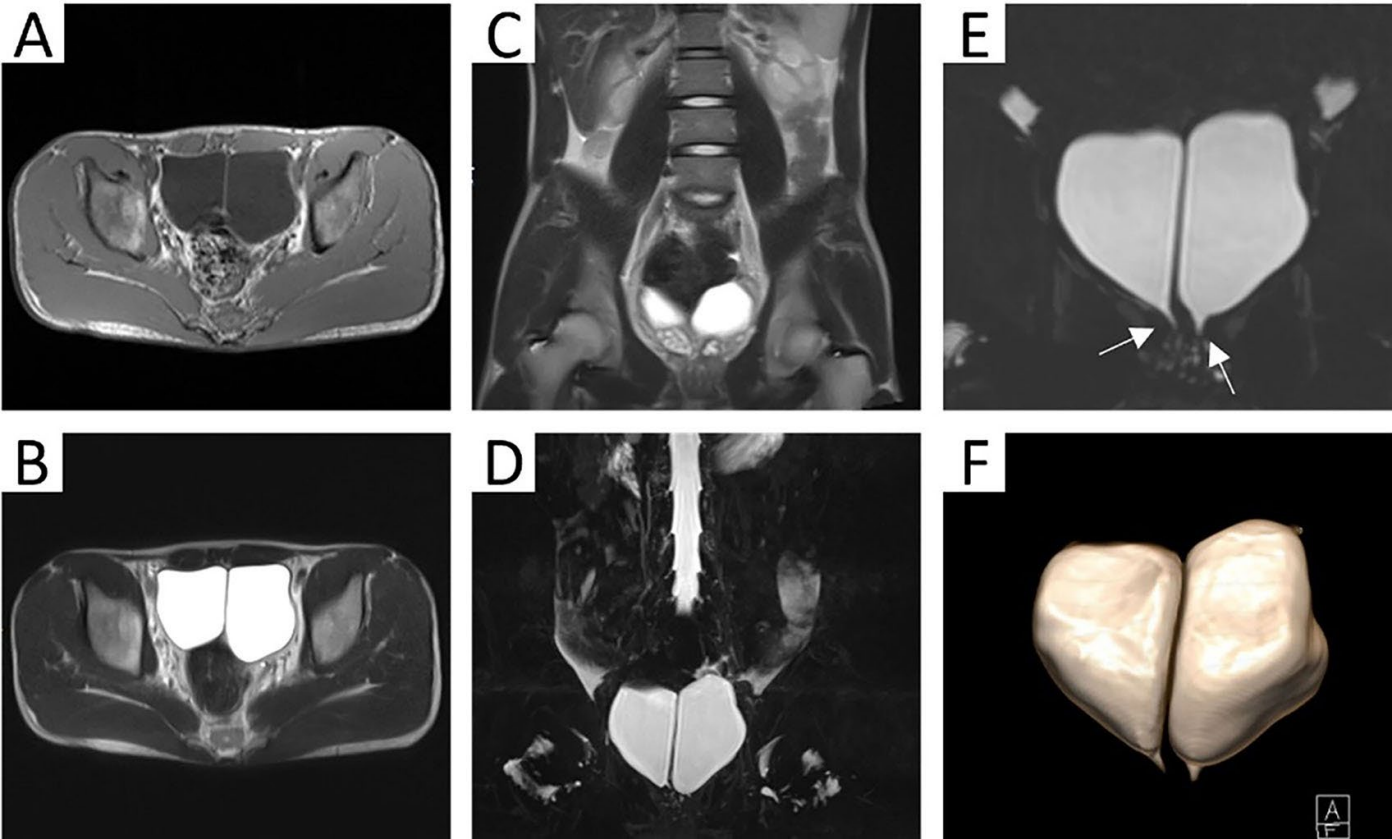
MRI: T1WI, T2WI cross-section and coronal plane: two chambers in the pelvic arranged left and right, the two chambers were separated by a sagittal septum, and septum shape and signal were similar to the bladder wall (A-C). MRU: two bladders were connected to the bilateral posterior urethra respectively (D-E). 3D: two half-bladders and two posterior urethras (F)
